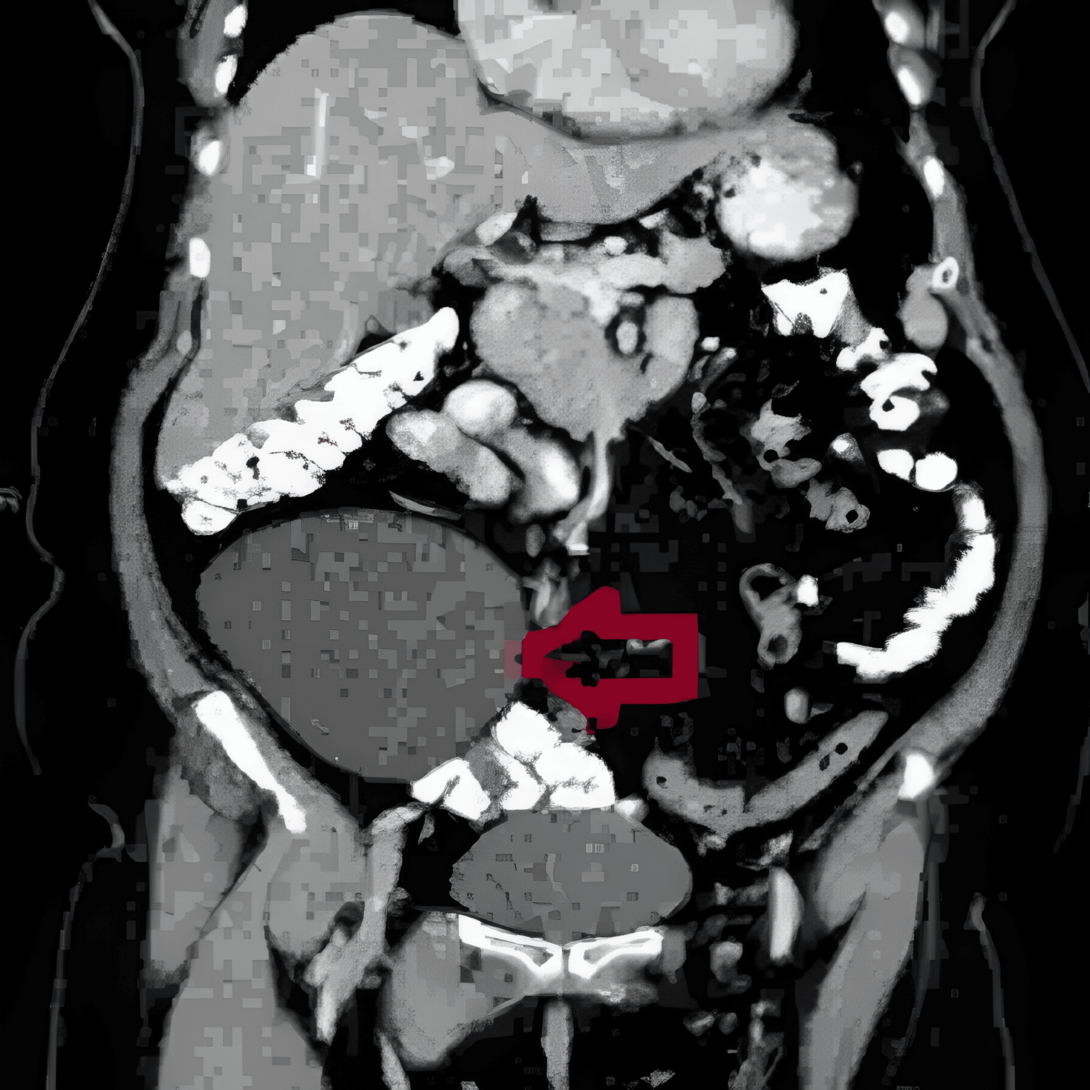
Coronal view of CECT demonstrating the mesenteric cyst (arrow) CECT, contrast-enhanced CT
